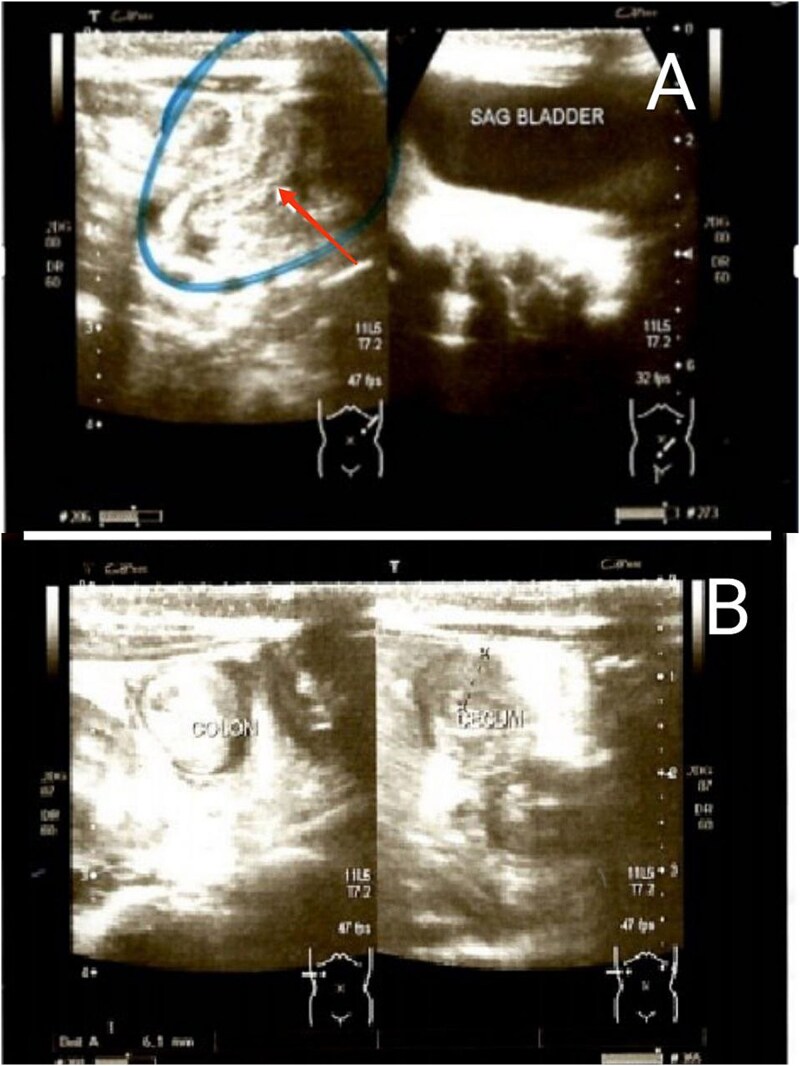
Ultrasonography findings (A) ultrasonography of the left hypochondriac and inguinal regions showing dilated small bowel loops (red arrow). (B) Ultrasonography of the right hypochondriac region demonstrating a high-positioned cecum with markedly thickened and edematous walls (approximately 6 mm).
